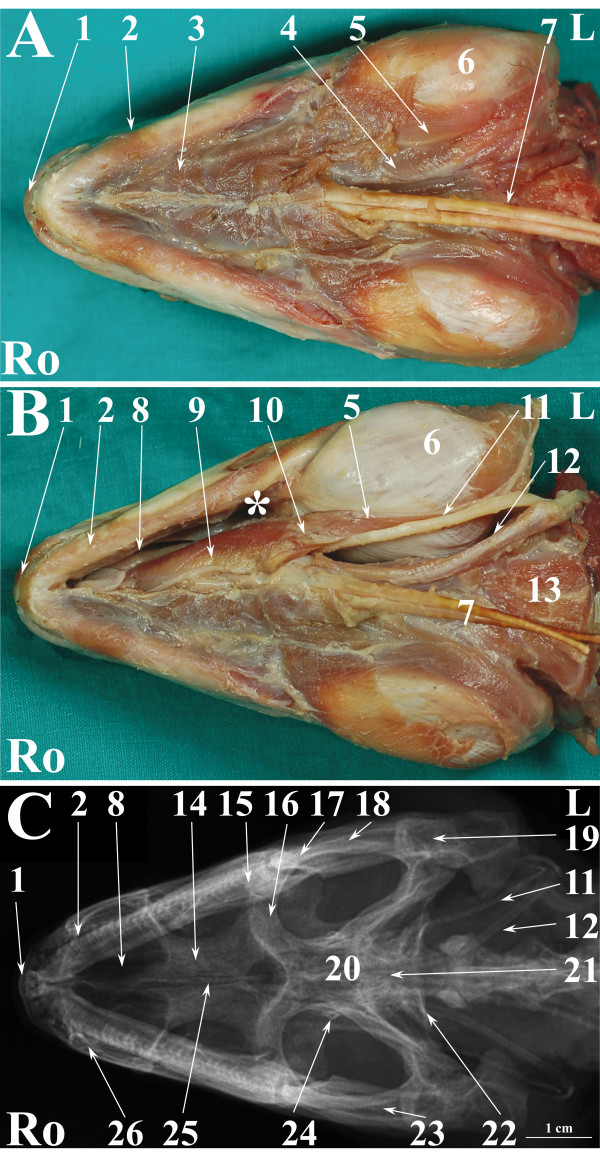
Normal radiographic anatomy of the head of the green iguana in VD projection. A. Ventral view in a superficial plane of stratigraphic dissection (only skin was removed) of the head of an iguana. B. Ventral view of stratigraphic dissection after removal of Musculus constrictor colli and Musculus intermandibularis (lower part of the image) and a deeper plane of dissection (upper part of the image) of the head of an iguana (deeper plane of dissection is labelled with * on the image). C. VD radiographic projection of the head of an iguana. Ro is rostral, L is left.1. Premaxillary bone; 2. Dentary bone; 3. Musculus intermandibularis; 4. Musculus geniohyoideus; 5. Musculus hyoglossus; 6. Musculus pterygoideus typicus; 7. Ceratobranchial 1 process of the hyobranchial skeleton; 8. Vomer bone; 9. Musculus genioglossus; 10. Tendinous band; 11. Ceratohyal process of the hyobranchial skeleton; 12. Ceratobranchial 2 process of the hyobranchial skeleton; 13. Musculus omohyoideus; 14. Palatine bone; 15. Ectopterygoid bone; 16. Pterygoid bone; 17. Jugal bone; 18. Postorbital bone; 19. Articular bone + quadrate bone; 20. Parietal bone; 21. Basioccipital bone; 22. Paraoccipital process of otooccipital bone; 23. Adductor fossa; 24. Epipterygoid bone; 25; Interpterygoid vacuiti; 26. Maxillary bone.
